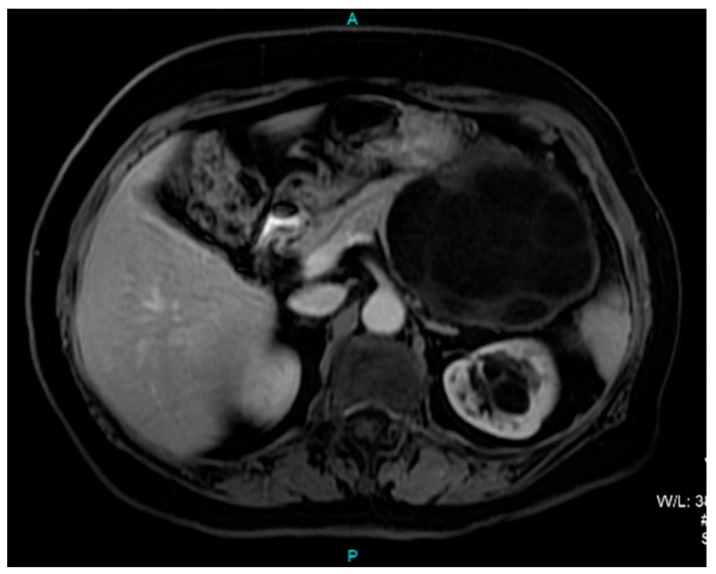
Axial T1-weighted postcontrast MRI image shows a predominantly hypointense cystic lesion in the region of the pancreatic tail without any solid component.
